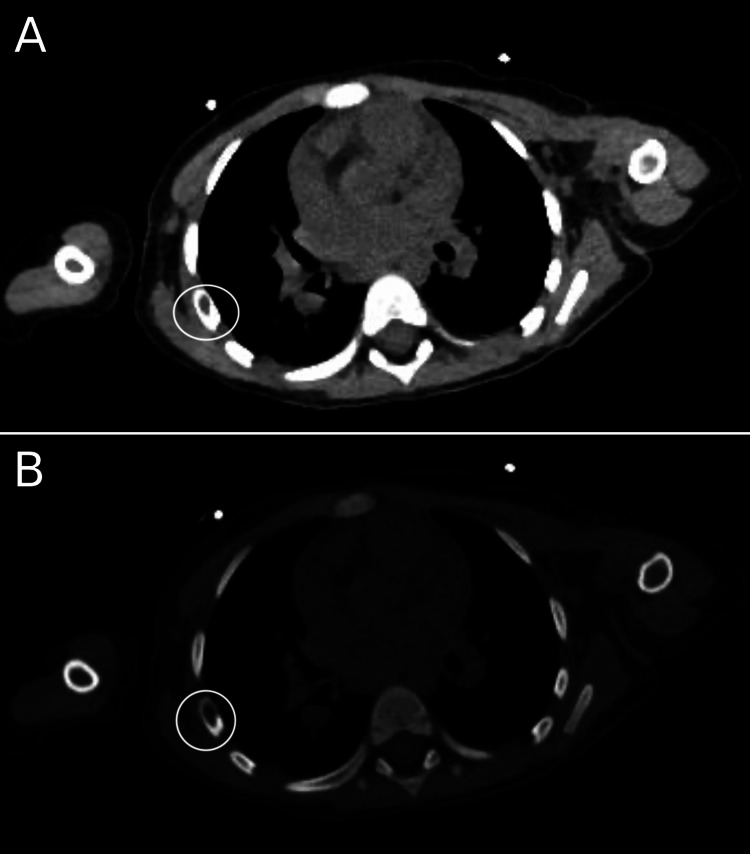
CT scan showing a lytic lesion in the right fifth rib.
